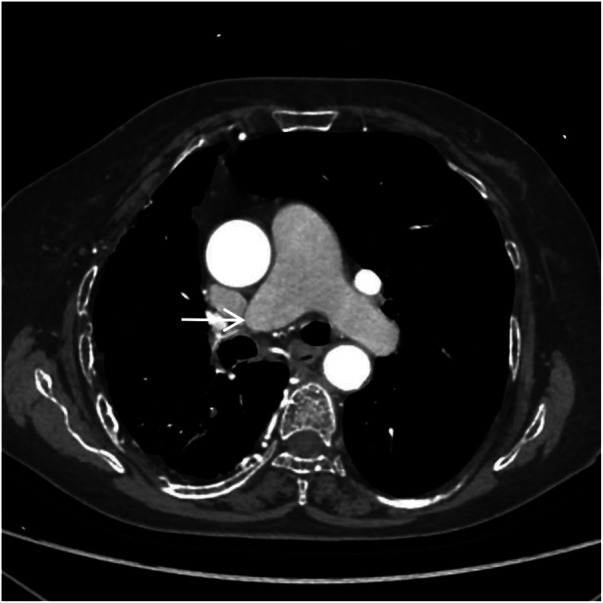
CTA axial image shows absence of the distal right pulmonary artery, with a rounded and blunt stump (white arrow). No abnormal density shadows are observed within the main pulmonary artery and the proximal segments of the left and right pulmonary arteries.
